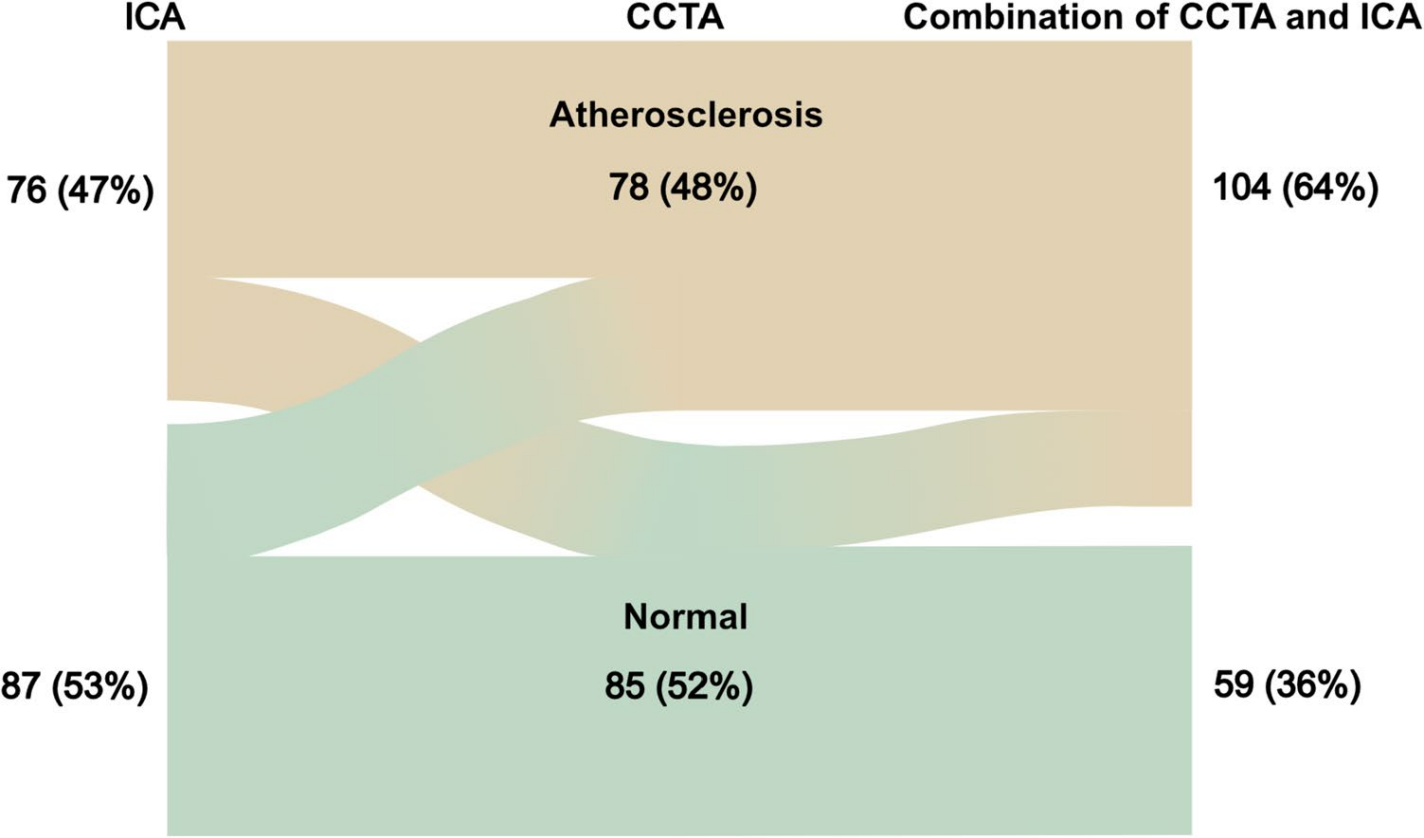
Coronary atherosclerotic segments were identified using ICA or CCTA separately, as well as in combination at the patient level, and are presented as numbers with corresponding percentages. *CCTA* coronary computed tomography angiography, *ICA* invasive coronary angiography
